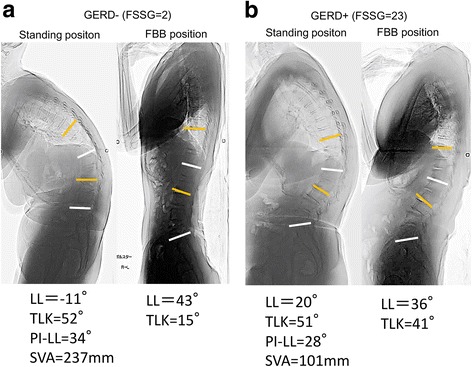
Representative cases with or without severe gastroesophageal reflux disease symptoms: Frequency Scale for Symptoms of GERD score of 2 (a) or 23 (b). The white line denotes lumbar lordosis. The yellow line denotes thoracic lumbar kyphosis a Lateral standing radiograph showing global sagittal malalignment due to a − 11° lumbar lordosis and a 51° thoracic lumbar kyphosis. Lateral radiograph obtained in the fulcrum backward-bending position showing thoracic lumbar kyphosis reduced to 15°. b Lateral standing radiograph showing a − 20° lumbar lordosis and global sagittal malalignment due to a 51° thoracic lumbar kyphosis. Lateral radiograph obtained in the fulcrum backward-bending position showing a 41° thoracic lumbar kyphosis that is not flexible.
